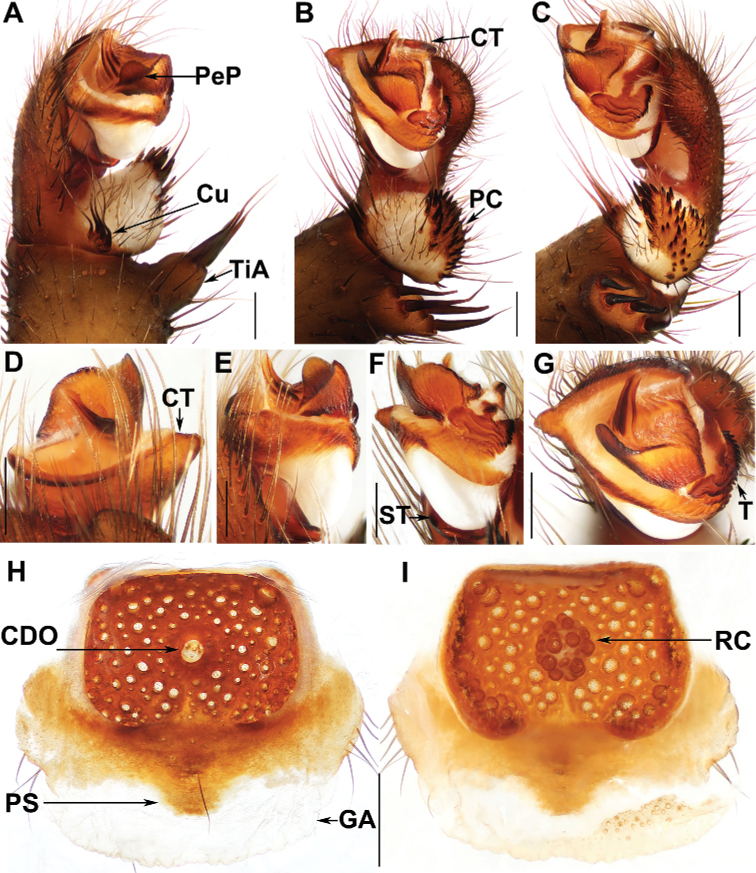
Male palp and female genitalia of *Liphistiushatyai* Zhan & Xu, sp. nov. A palp, prolateral view B palp, ventral view C palp, retrolateral view D–G palp, distal views H vulva, dorsal view I vulva, ventral view A–G XUX-2017-492 (holotype) H, I XUX-2017-493. Abbreviations used: CDO = central dorsal opening; GA = genital atrium; PS = posterior stalk; RC = receptacular cluster; CT = contrategulum; Cu = cumulus; PC = paracymbium; PeP = paraembolic plate; ST = subtegulum; T = tegulum; TiA = tibial apophysis. Scale bars: 0.5 mm.
